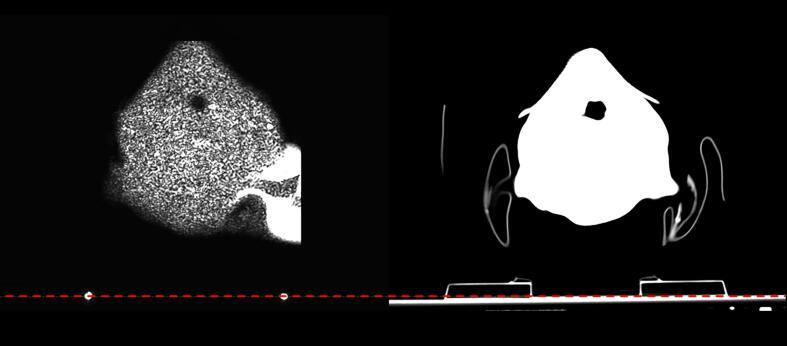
To the left, an image slice from TSE FLAIR MR sequence where the couch top markers can be seen. The corresponding CT image slice is shown to the right.
